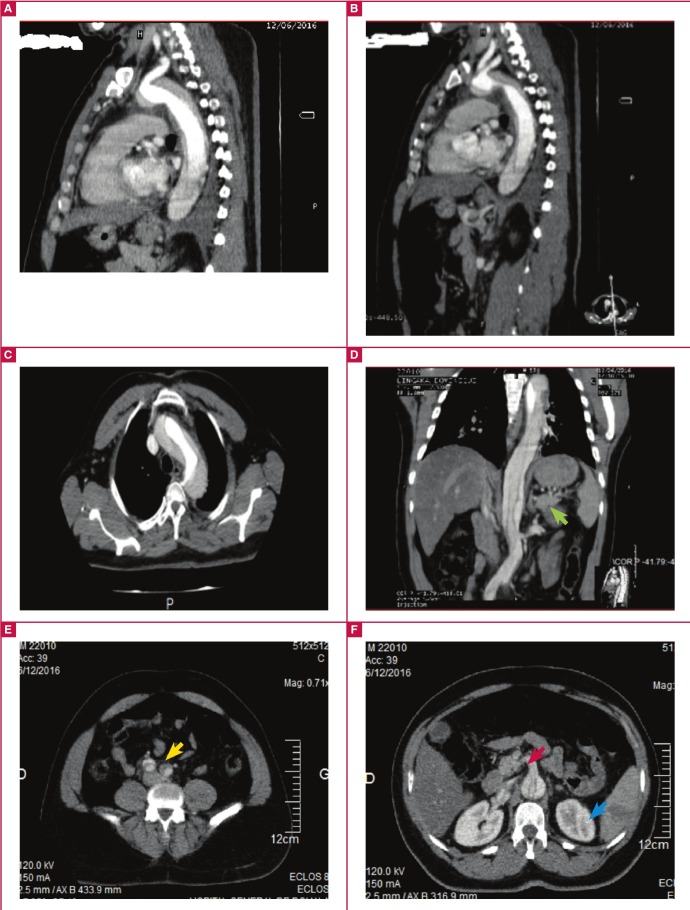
Contrast-enhanced CT angiogram of the thorax showing aortic dissection extending to the left renal (green arrow), iliac (yellow arrow) and superior mesenteric (red arrow) arteries and causing splenic infarction (blue arrow).
